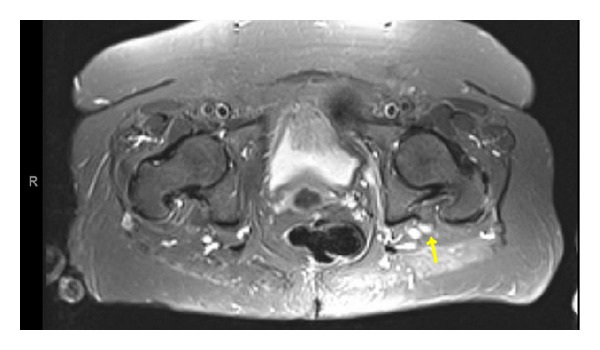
Axial MRI-STIR of the pelvis without contrast. There is asymmetric high T2 signal and enlargement of the left sciatic nerve as seen on axial STIR image localized to pelvic region (marked by yellow arrow).
